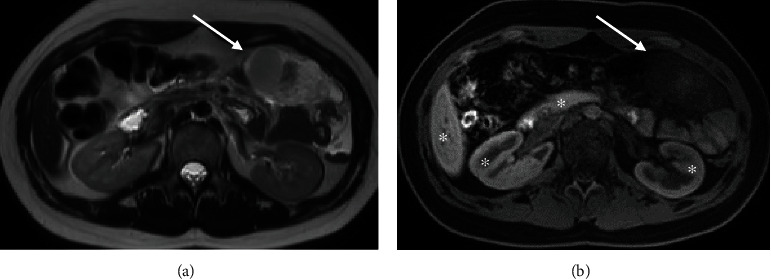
Follow-up noncontrast MRI 18 hours after the initial MRI study. Axial T2-weighted image shows an anterior shift in position of the lesion which remains heterogenous with predominantly hyperintense signal (arrow in (a)). There are no new areas of significant signal loss to suggest deoxyhemoglobin formation. Corresponding axial T1 fat sat image shows no retention or layering of contrast from the prior contrast-enhanced MRI exam (arrow in (b)). There remains no evidence of hyperintense signal to suggest methemoglobin formation. Note residual contrast enhancement of the liver, pancreas, and kidneys (asterisks in (b)).
